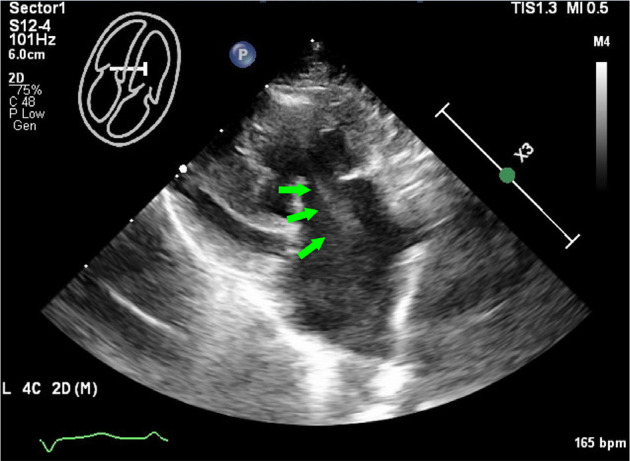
B-mode echocardiographic image showing spontaneous echocardiographic contrast (green arrow) in the left atrium in the left apical four-chamber view
